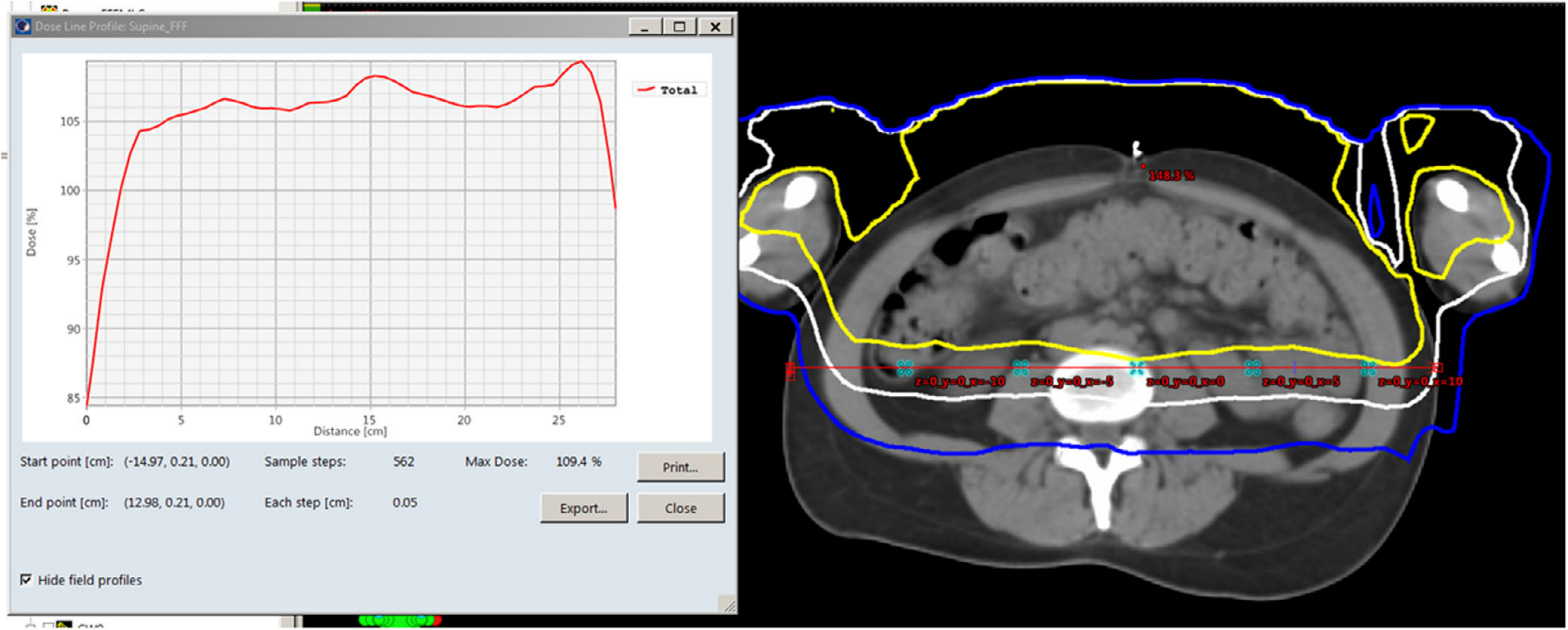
A screen capture from Eclipse of an axial CT slice of a patient at the level of the umbilicus with a supine open field 6 MV FFF VMAT TBI plan applied. On the right is the CT image with the 110% isodose level in yellow, the 100% isodose level in white, and the 90% isodose level in blue. The mid‐plane red line on the CT image indicates the location of the dose profile, with the profile itself presented on the left. The crosses along the mid‐plane red line on the CT image are reference points. FFF, flattening filter free; TBI, total body irradiation; VMAT, volumetric modulated arc therapy.
